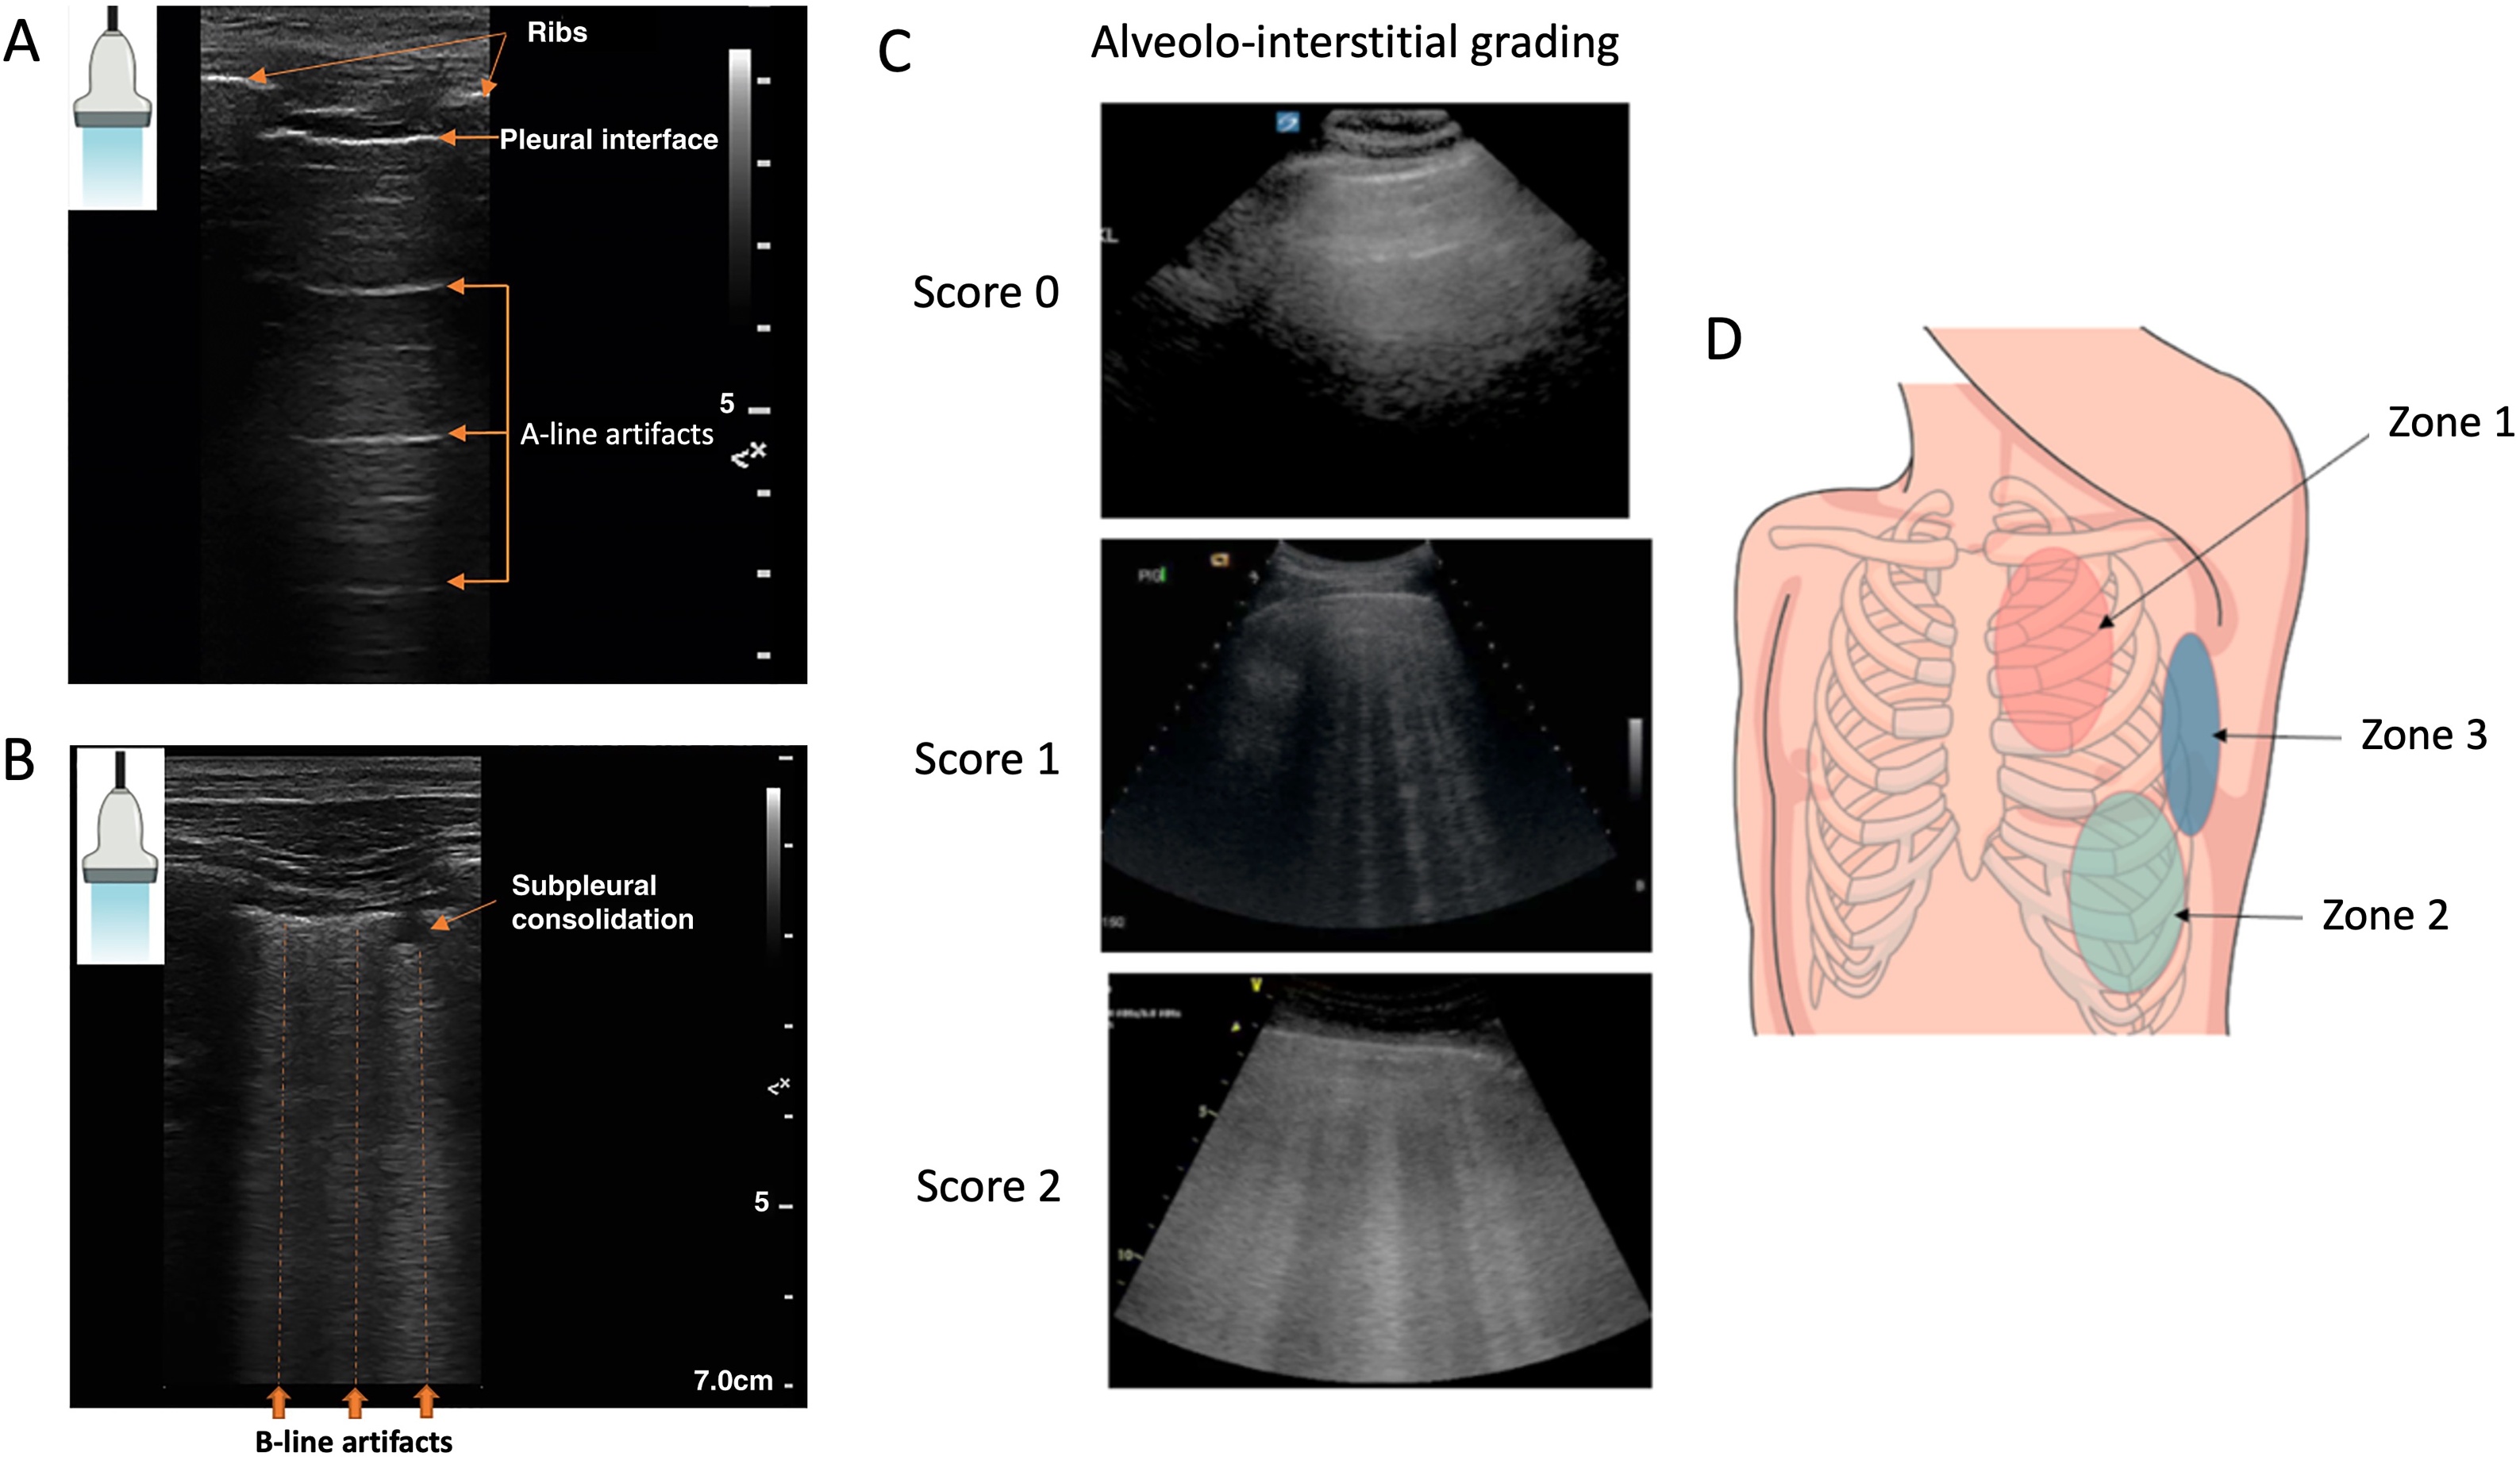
Lung ultrasound for evaluation of congestion. (A) Normal appearance including rib shadows, pleural interface, and horizontal a-line artifacts. (B) Presence of alveolar-interstitial syndrome associated with the appearance of at least 3 vertical b-line artifacts that arise from the pleura. (C) Lung ultrasound scoring system to quantify loss of lung aeration (Score 0 if A-lines or ≤ 2 B-lines; Score 1 if ≥ 3 distinct B-lines; Score 2 if presence of coalescent B-lines). (D) Three per side scanning protocol.
